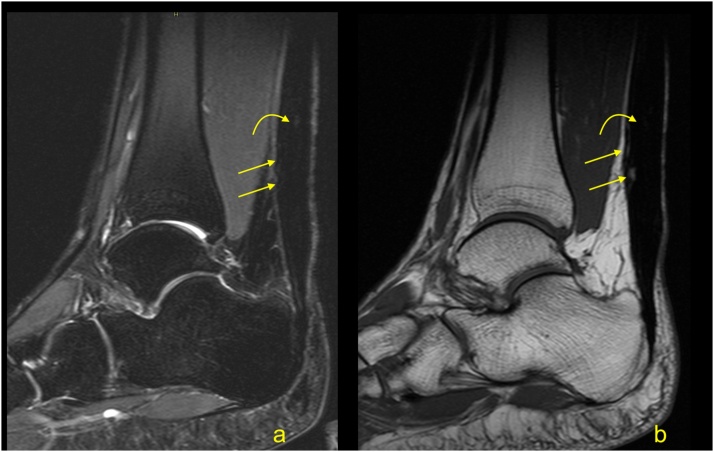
Degeneration of the Achilles tendon. MRI revealed focal fat degeneration (straight arrow), which on T1-weighted imaging shows a higher signal and is suppressed on T2-weighted imaging with fat suppression. There are also the focal fluid lesions (curved arrow). A-T2-weighted with fat suppression, b- T1-weighted.
